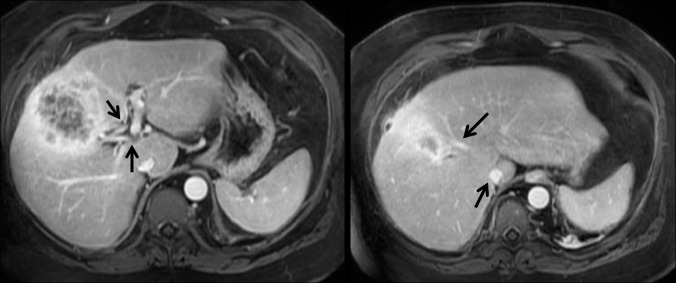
Magnetic resonance imaging (MRI) with no portal or hepatic vessel spread (arrows)
